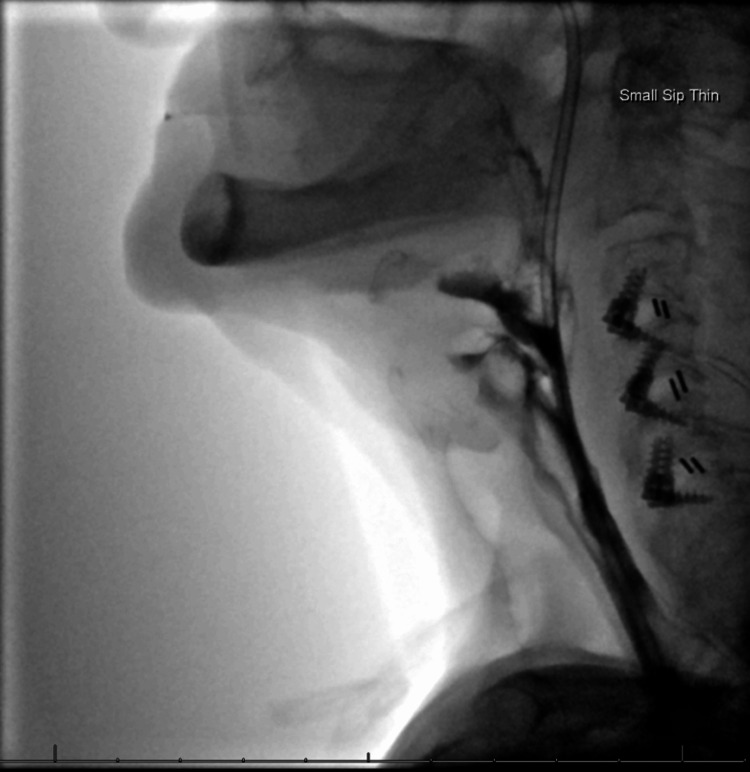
VFSS performed three days postoperatively demonstrating contrast passage through the pharynx into the oesophagus without evidence of aspiration or airway penetration on this swallow sequence. Original X-ray image from Royal Hobart Hospital, Australia, 2025, reproduced with permission from Royal Hobart Hospital Radiology Department VFSS: Videofluoroscopic swallow study
